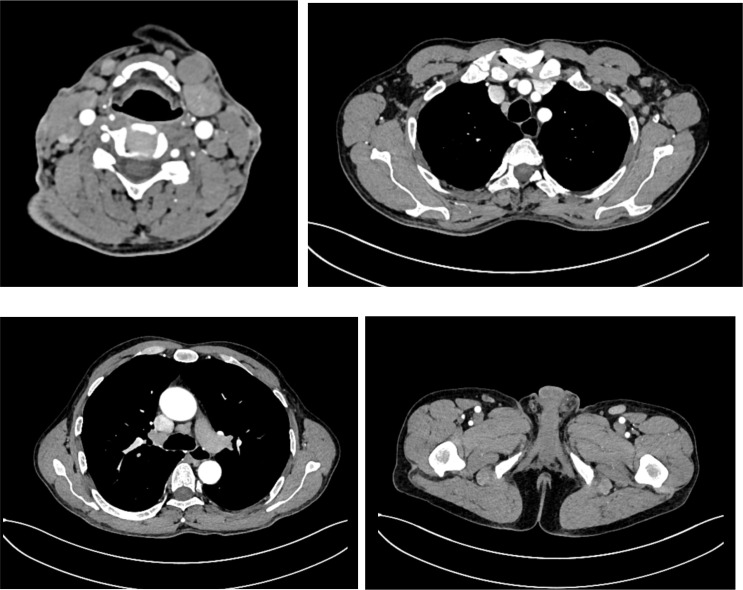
CT scan (January 16, 2024) showing multiple enlarged cervical lymph nodes, some forming confluent masses, along with enlarged nodes in the bilateral axillae, supraclavicular and infraclavicular regions, mediastinum, retroperitoneum, and bilateral inguinal regions.
